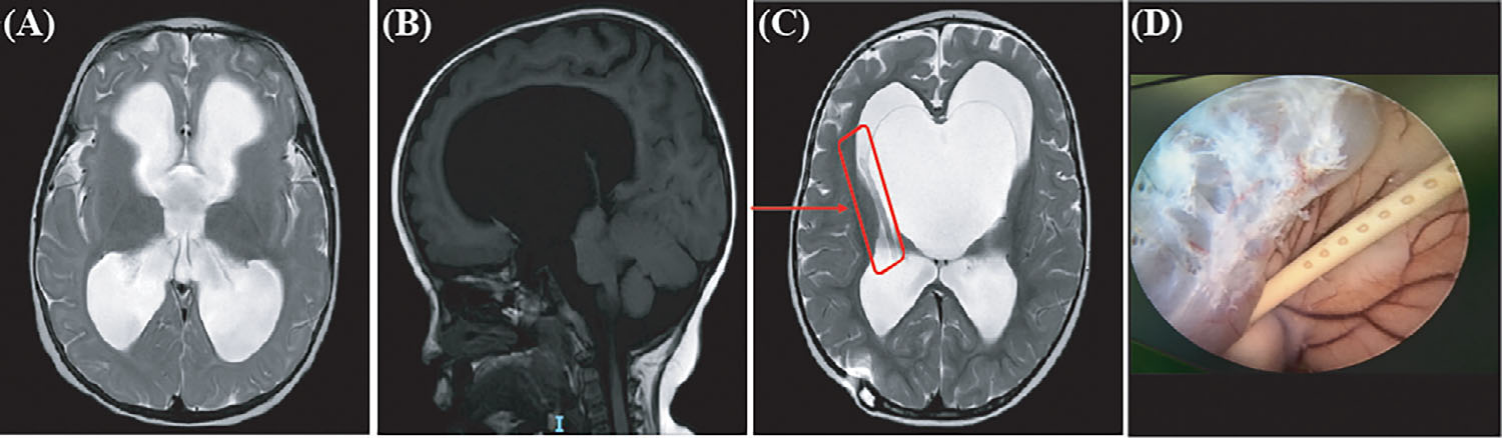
Images of patient 1. (A) Magnetic resonance imaging (MRI)‐T2WI sagittal image and (B) coronal image showing hydrocephalus prior to ventriculoperitoneal shunt surgery; (C) MRI‐T2WI image shows the ventricular tube (red arrow) closely adhering to the ventricular wall, with a large cyst visible; (D) During ventriculostomy, the drainage tubes and cysts are visible.
